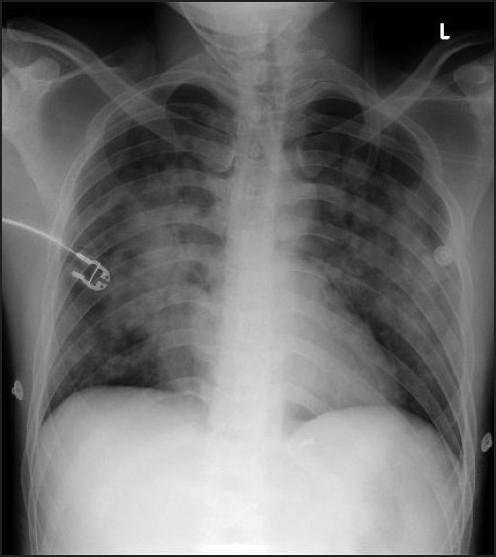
Second post-operative day chest X-ray, showing prominent mid-zone perihilar infiltrates
